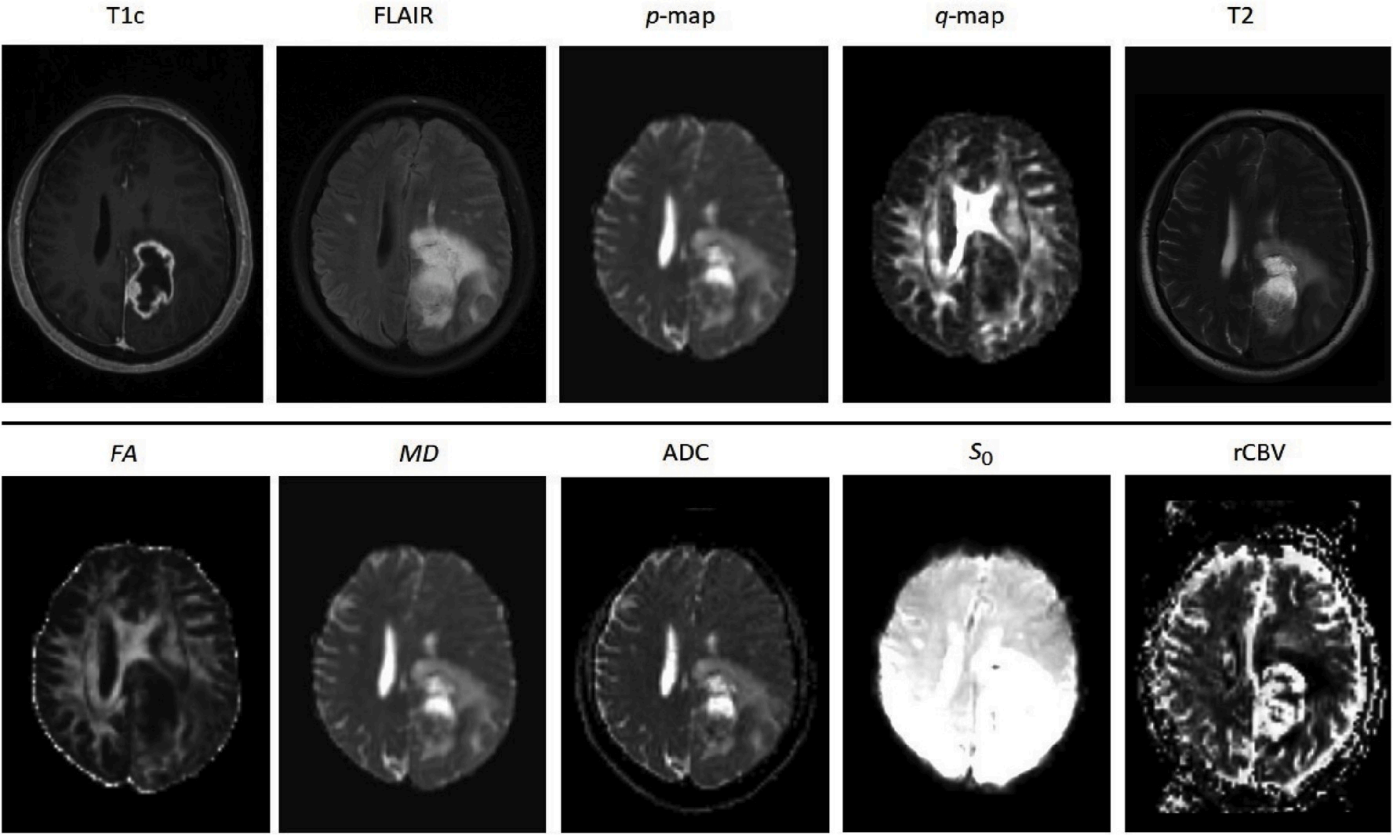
Ten different MRI modalities used in this study which consist of anatomical (T1c, FLAIR and T2), DTI (*p*, *q*, FA and MD, ADC) and PWI images ($S_{0}$ and rCBV).
